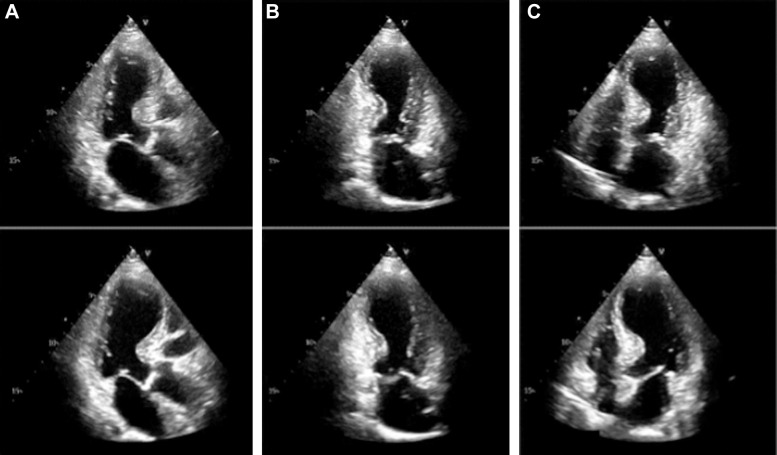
Bedside Echocardiography (Cardiac Point-of-Care Ultrasound) During the Acute Phase of Takotsubo Syndrome The upper row shows left ventricle images during systole, the lower row shows images during diastole. (А) Apical 3-chamber view, (В) apical 2-chamber view, and (С) apical 4-chamber view. Ejection fraction was reduced to 47%, and hypokinesis of the left ventricle apex, apical segments of the anterior, inferior walls, and the interventricular septum was observed.
